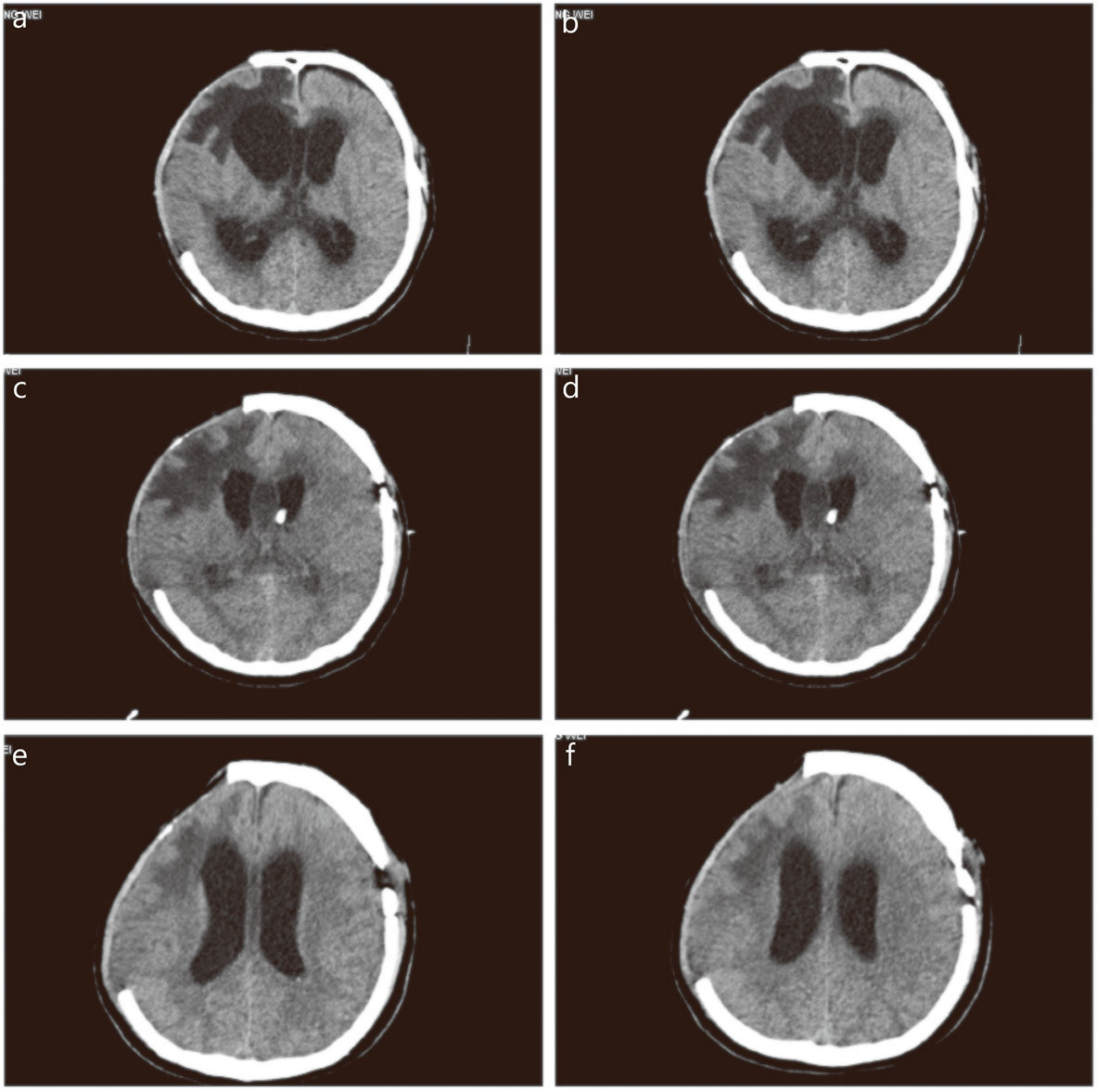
Brain CT after admission in patient with intracranial *A. baumanni* infection. a. Before the treatment on July 3, large low-density shadows were seen in the right frontotemporal parietal lobe; b. Before the treatment on July 3, large low-density shadows appeared in the bilateral lateral ventricles and the third ventricle dilated; c. During the treatment on July 16, bilateral lateral ventricle and the third ventricle were dilated, and hydrocephalus was better than before; d. During the treatment on July 16, a free tube shadow was seen through the left frontal bone to the anterior horn of the left ventricle; e. After treatment on August 2, the original free tube shadow was removed; f. After treatment on August 2, bilateral lateral ventricular hydrocephalus was observed
